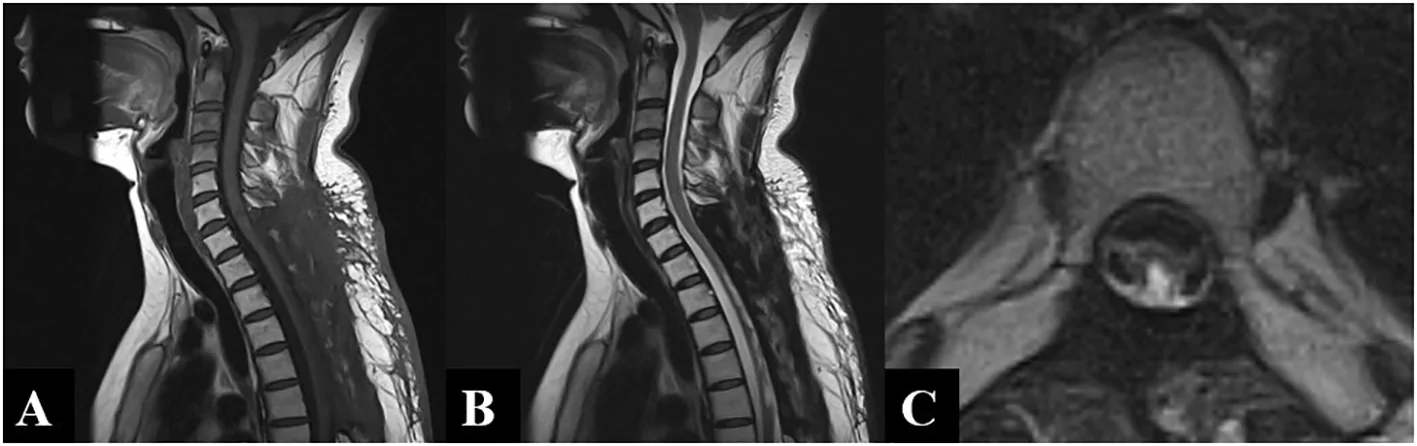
Six-month follow-up magnetic resonance imaging of the cervicothoracic spinal cord. (A) Sagittal T1-weighted image. (B) Sagittal T2-weighted image. (C) Axial T2-weighted image. Images demonstrate irregular spinal cord thinning and cystic–gliotic postoperative changes at T1–T5, without definite MRI evidence of disease progression at the time of examination.
